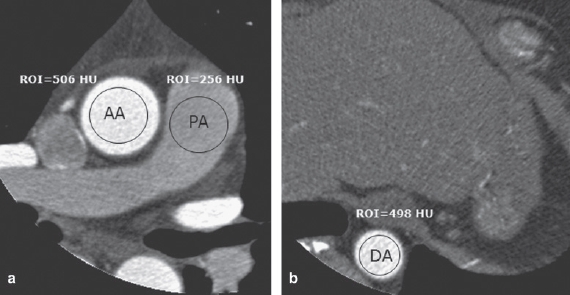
Axial images of coronary CT angiography in the same patient as in Fig. 1 are shown. The attenuation numbers of the great arteries were 506 HU for the ascending aorta (AA), 256 HU for the main pulmonary artery (PA) (a), and 498 HU for the descending aorta (DA) at the level of the inferior margin of the heart as determined on axial images (b). The calculated consistency of contrast material was 8 HU.
